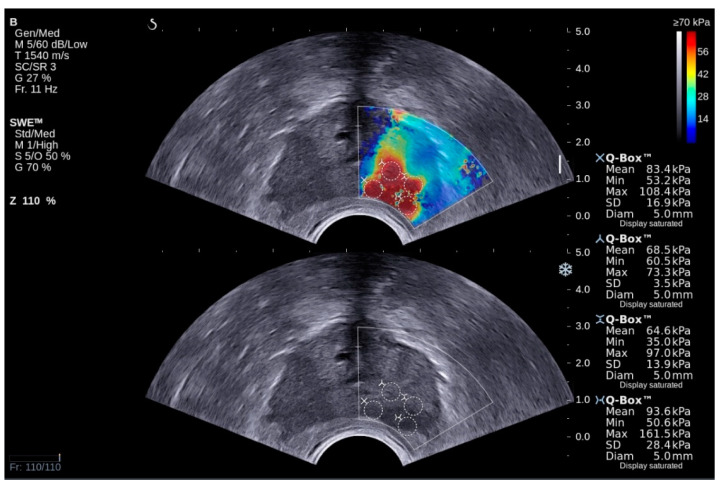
Concomitant transrectal visualization of the prostate bidimensional shear wave elastography (upper image) and grey-scale ultrasonography (lower image). Four regions of interest raise the suspicion of PCa (colored in red) at the level of the left prostatic lobe, and three of them were targeted during transrectal prostate biopsy. The pathology report has confirmed the PCa lesions in all three biopsy cores.
